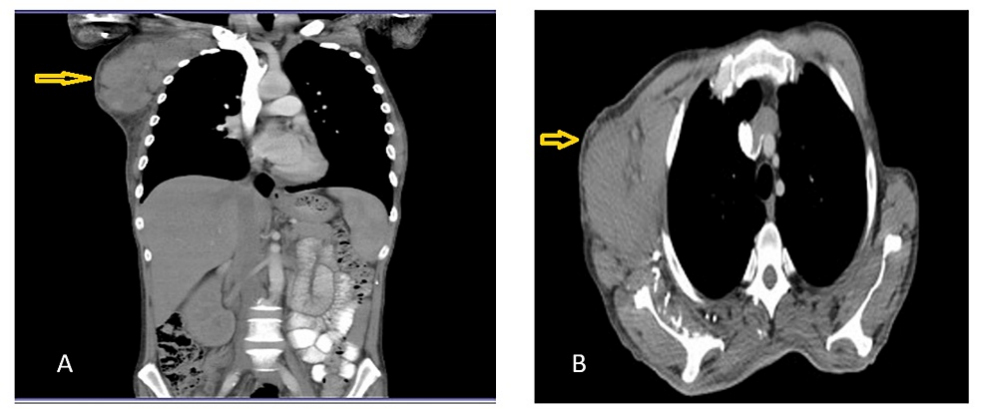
CT scan showing enlarged axillary and subpectoral lymph nodes on the coronal view (A) and transverse view (B).
